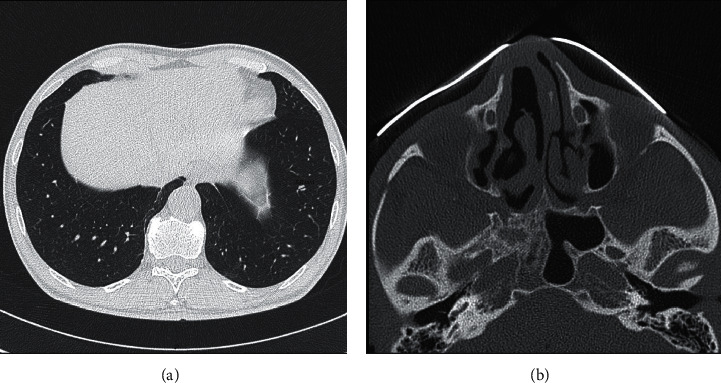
Computed tomography of lungs showing several micronodules (a) and that of sinus showing pansinusitis (b).
